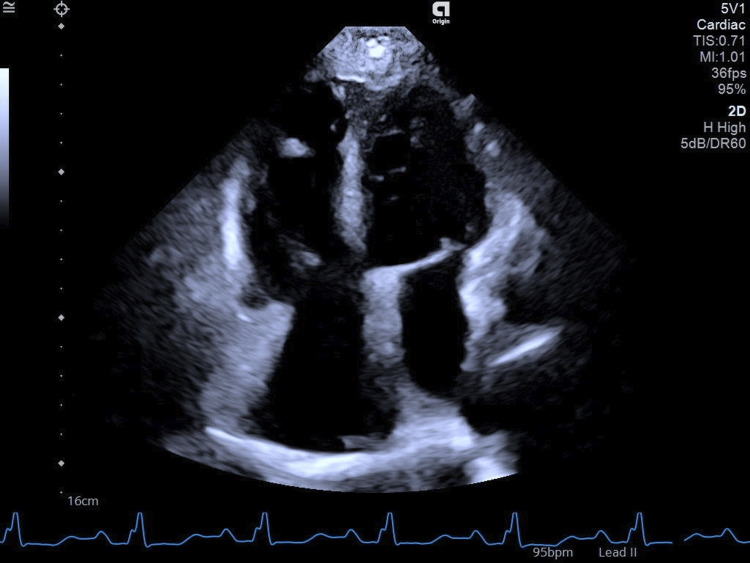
Transesophageal echo showing no vegetations over the mitral and tricuspid valves (ruling out infective endocarditis)
